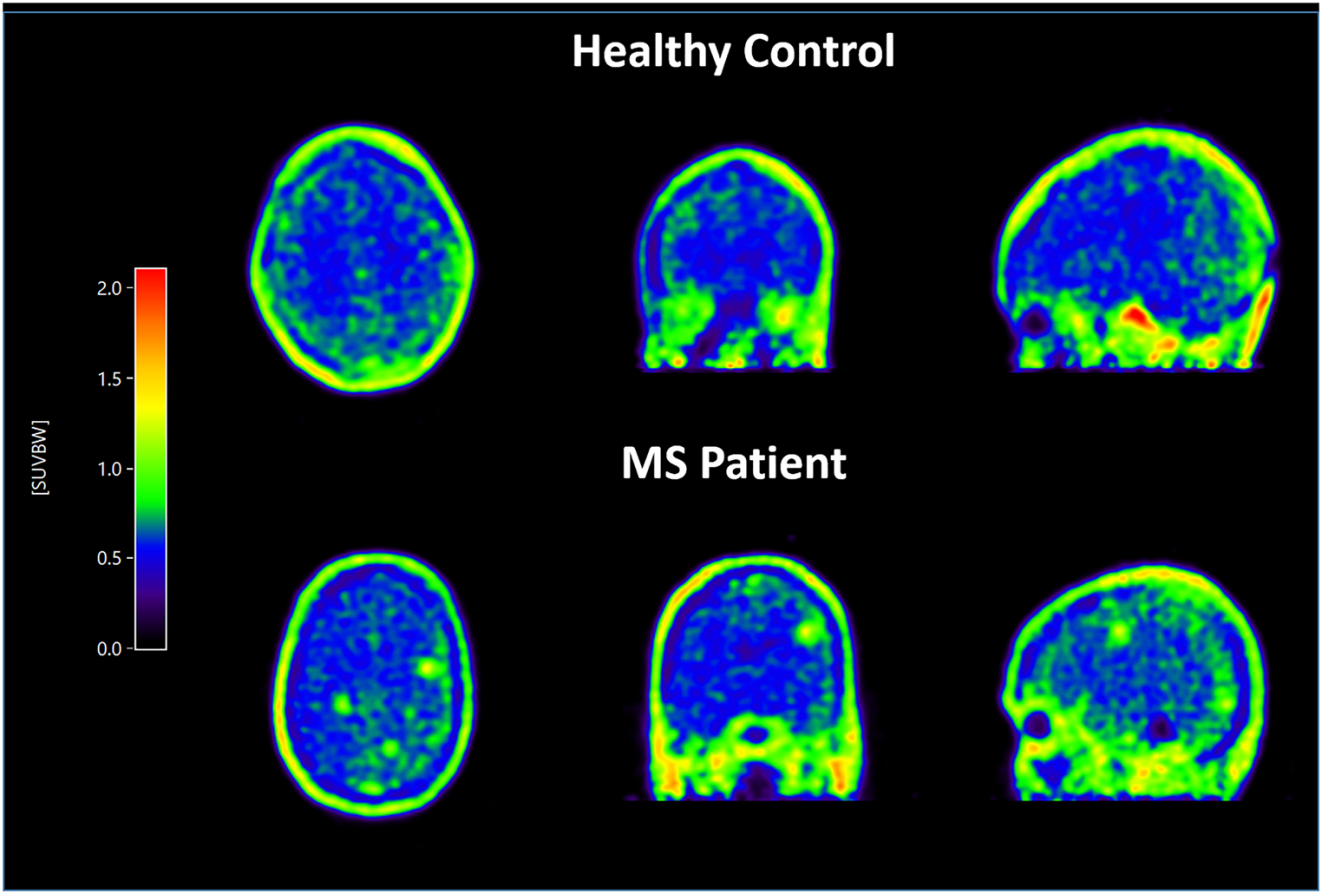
An SUV image of one healthy control and one patient
